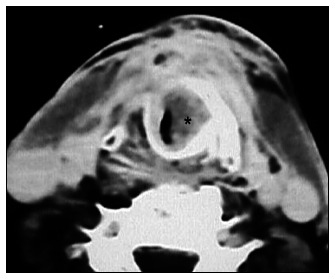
Axial computed tomography scan. Left vocal cord tumor (*). True-positive case.
